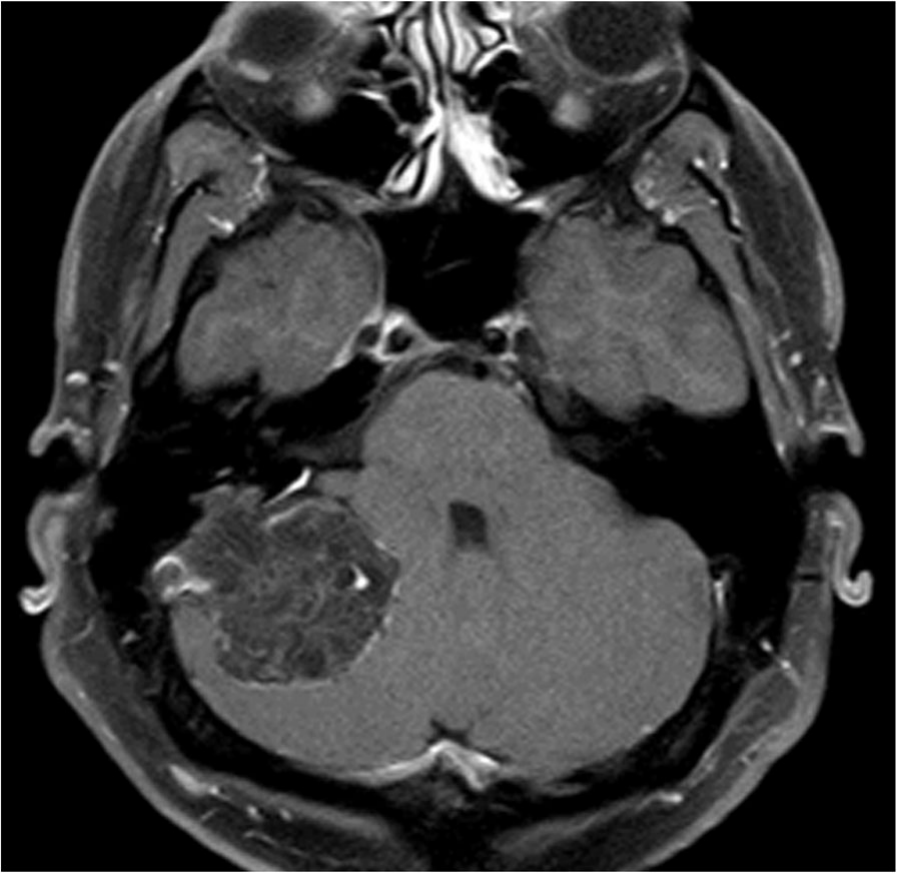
Endolymphatic sac tumor. Axial post-contrast T1-weighted MRI shows a bulky mass with mild heterogeneous enhancement that projects into the right posterior fossa from the posterior aspect of the right petrous bone
